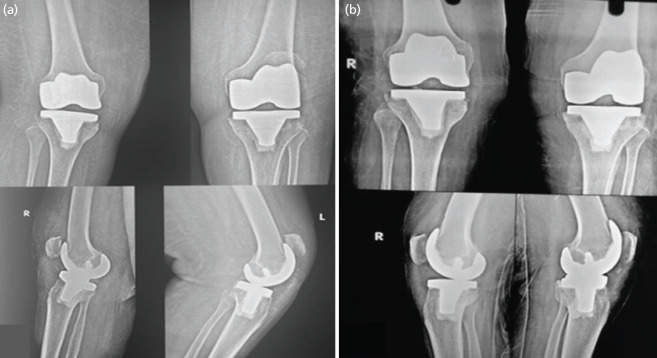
(a) Pre-operative radiograph suggestive of patella alta on the left side and medial joint line opening on the right knee, (b) post-operative radiograph.
